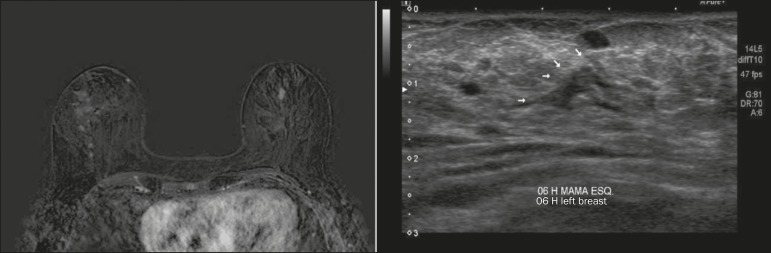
Fat-saturated T1-weighted sequences with digital subtraction, showing
